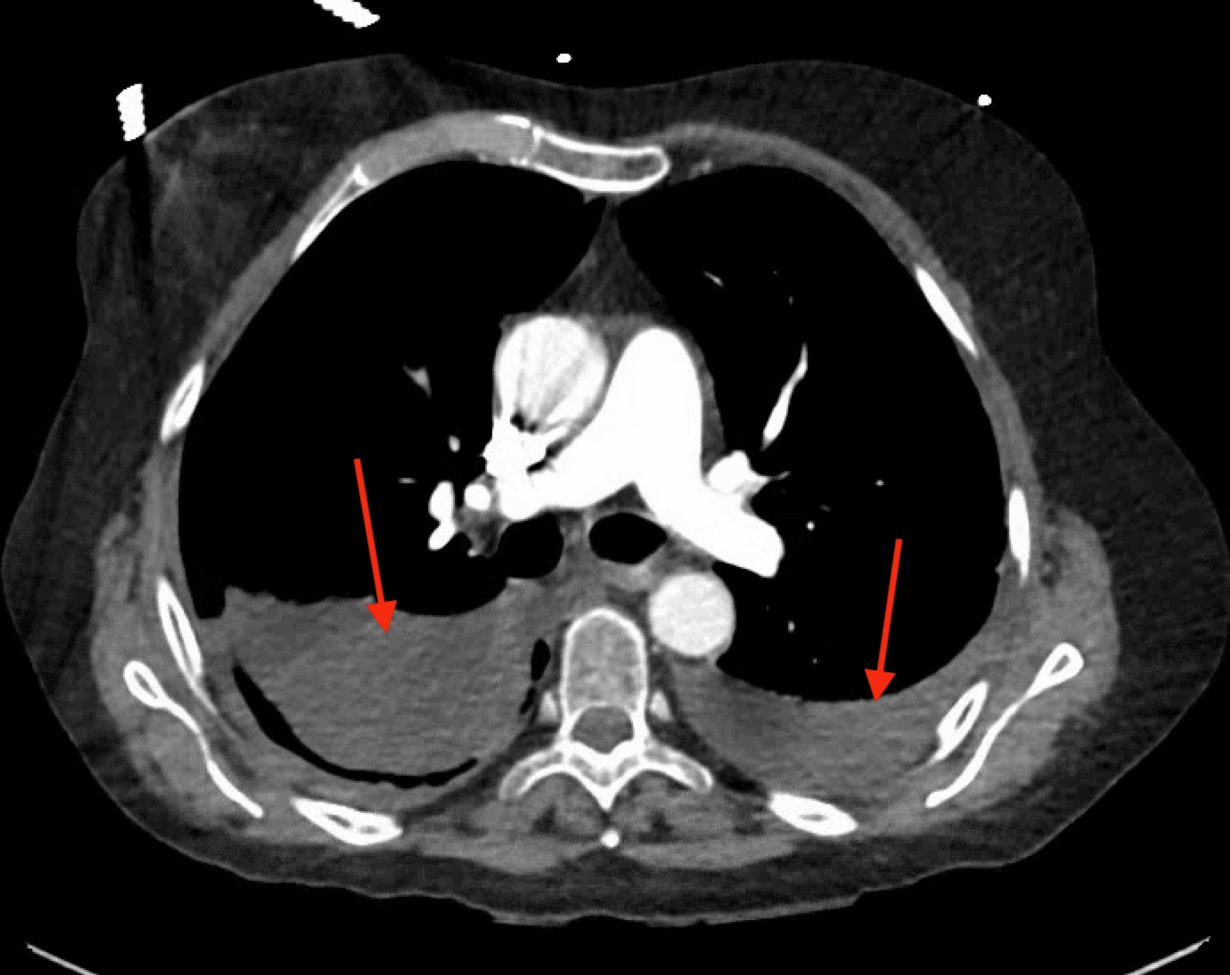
CT angiography of the chest shows bilateral pleural effusion, indicated by the red arrows.
